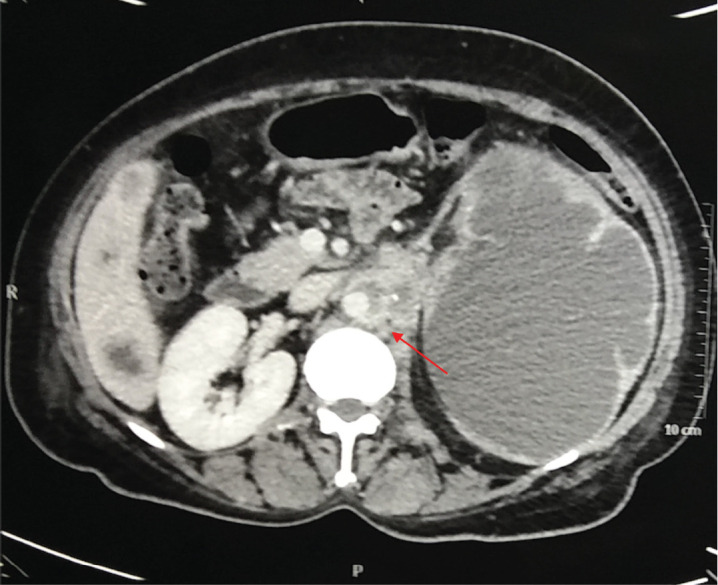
CT scan of the abdomen and pelvis (Transverse section) representing para aortic lymphadenopathy (red arrow).
